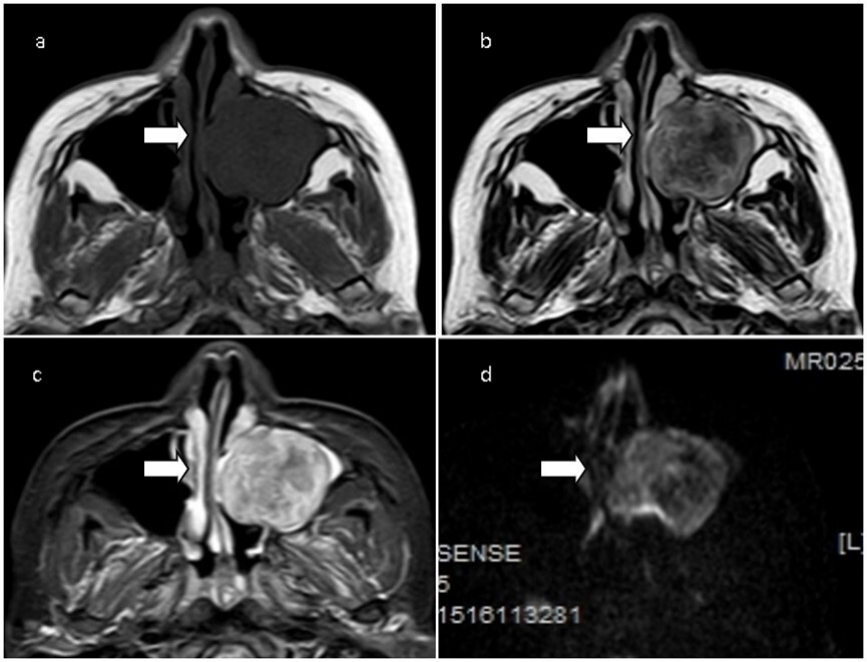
Transverse MR imaging of the nasal sinus demonstrated a solid mass occupying her left maxillary sinus(white arrows), measuring approximately 45 mm × 36 mm × 26 mm, and exhibiting hypointensity on T1WI(a), inhomogeneous hyperintensity on T2WI(b) and fat-suppression sequence(c), and moderate hyperintensity on DWI(d).
